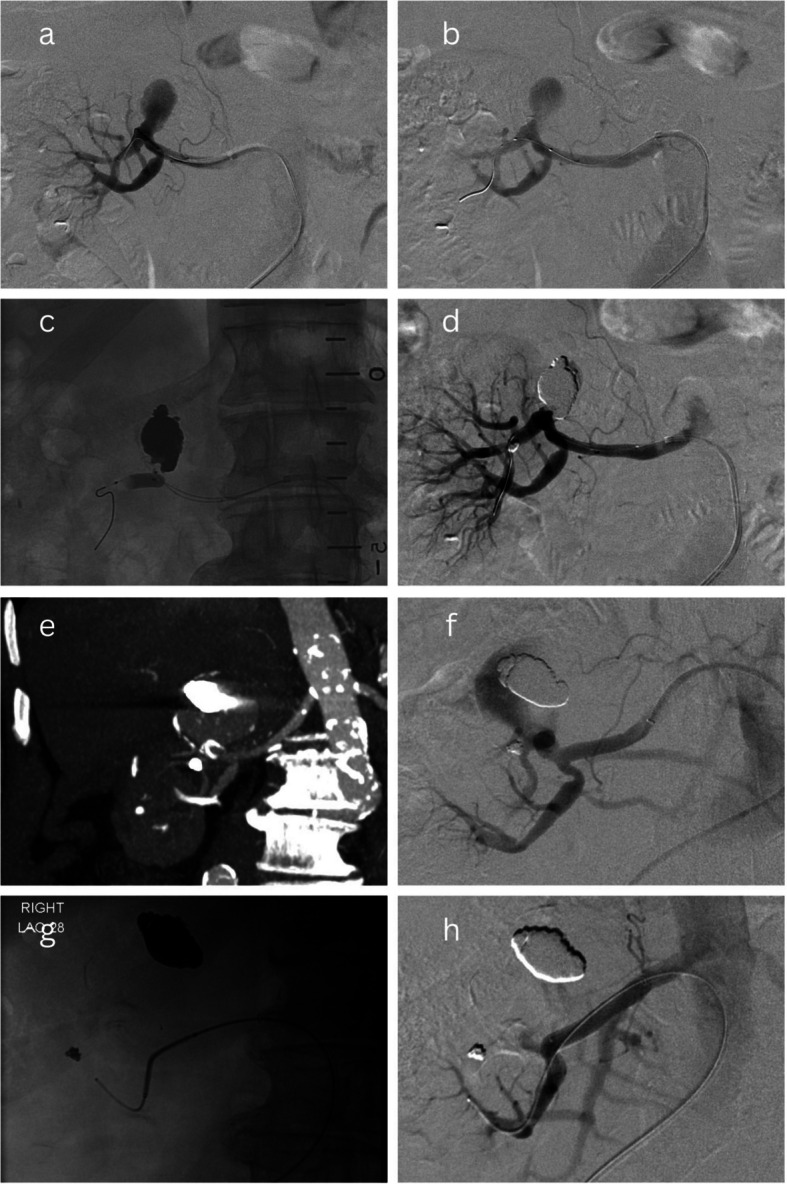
Reintervention case 3. A partially thrombosed 25 mm saccular Type 2 Rundback aneurysm (a) was treated endovascularly by manipulating a wire across the aneurysm into an efferent branch and positioning a balloon across the aneurysm’s origin (b). A separate microcatheter was placed in the aneurysm sac. The balloon was inflated and the sac was filled with Onyx until successful exclusion (c-d). CT imaging 8 years later incidentally demonstrated reperfusion and enlargement of the aneurysm (e). At reintervention, multiple attempts to cannulate the superior efferent branch were unsuccessful due to excessive tortuosity (f) and so a covered stent was placed from the main renal artery into the inferior segmental branch (g), successfully excluding the aneurysm but simultaneously sacrificing approximately 60% of renal parenchymal perfusion (h)
